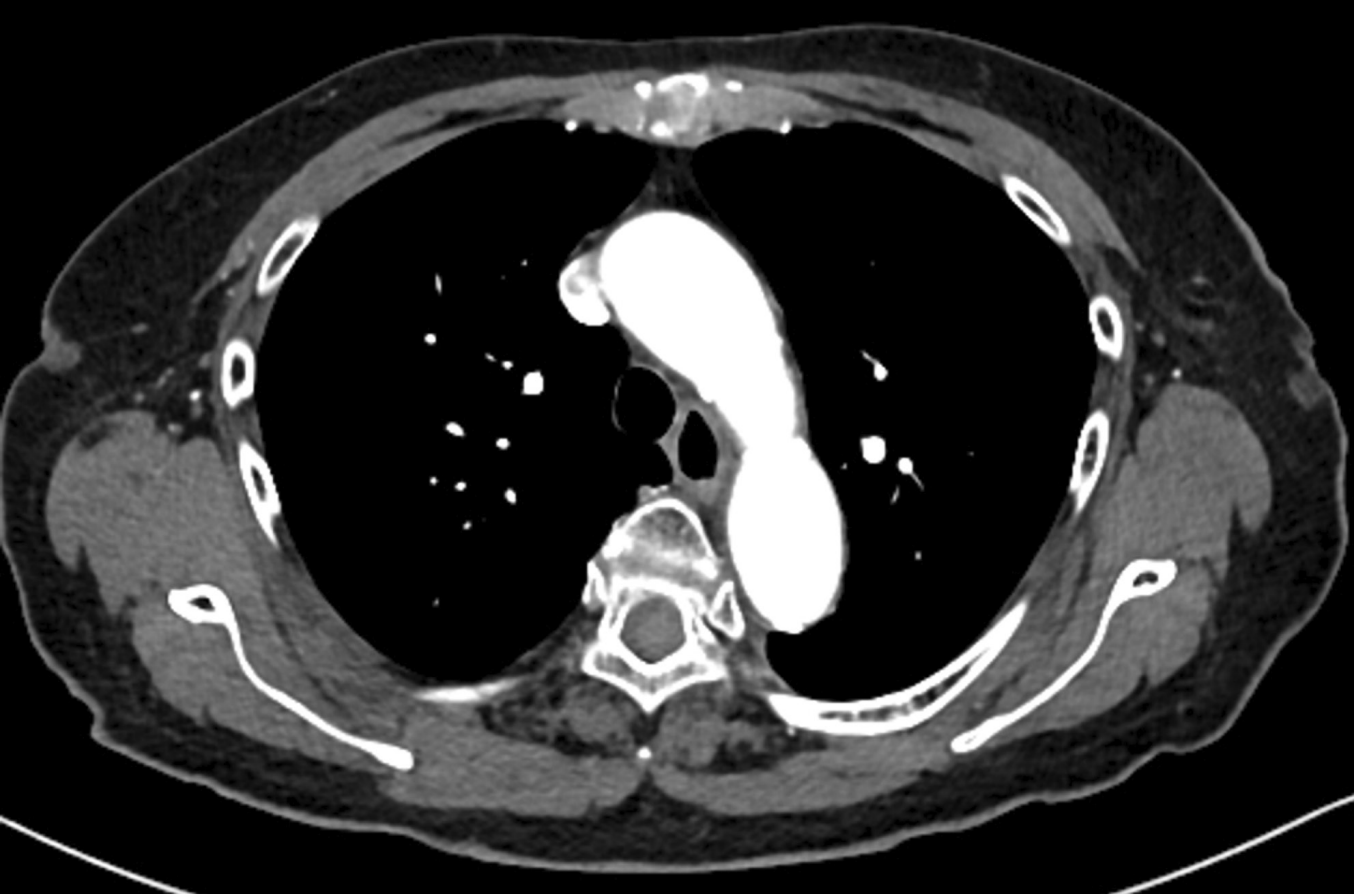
Contrast‐enhanced computed tomography. Contrast‐enhanced computed tomography show no artery dissection.
